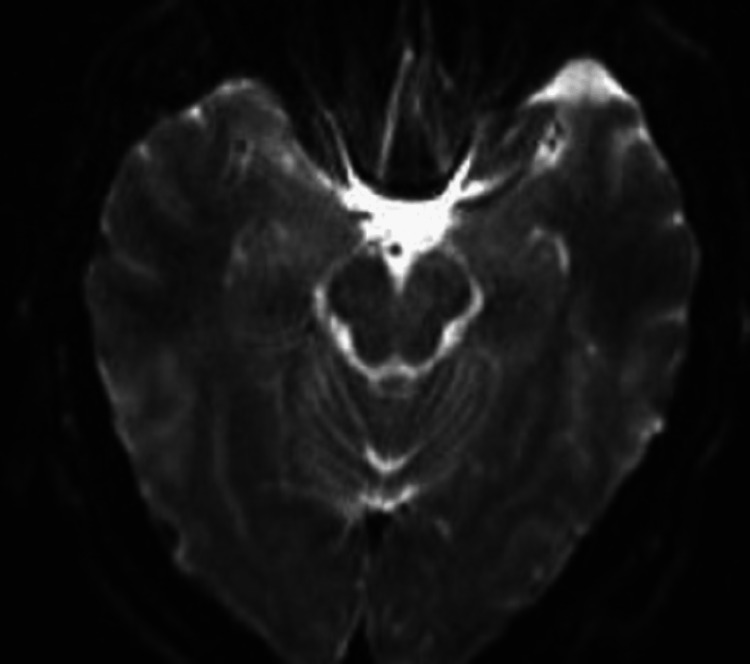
MRI T2-weighted sequence eight months after the episode
